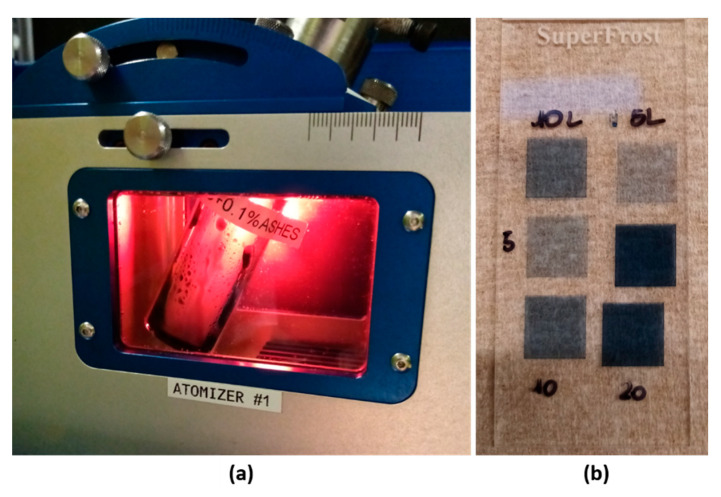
(a) Ultrasonic atomization in AJ^®^P setup and (b) pattern samples printed onto glass slides of poly(3,4-ethylenedioxythiophene)-poly(styrenesulfonate) (PEDOT:PSS) and nano-CA water-based dispersion with nano-CA concentrations of 0.1% wt/V_PEDOT:PSS_.
